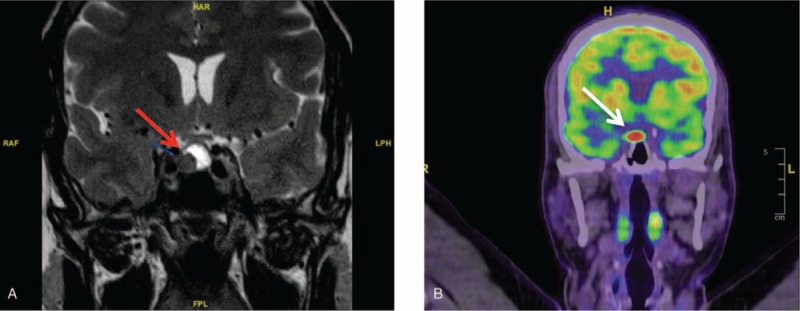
A and B: Pituitary adenoma discovered incidentally on PET-FDG and confirmed at MRI. MRI = magnetic resonance imaging.
